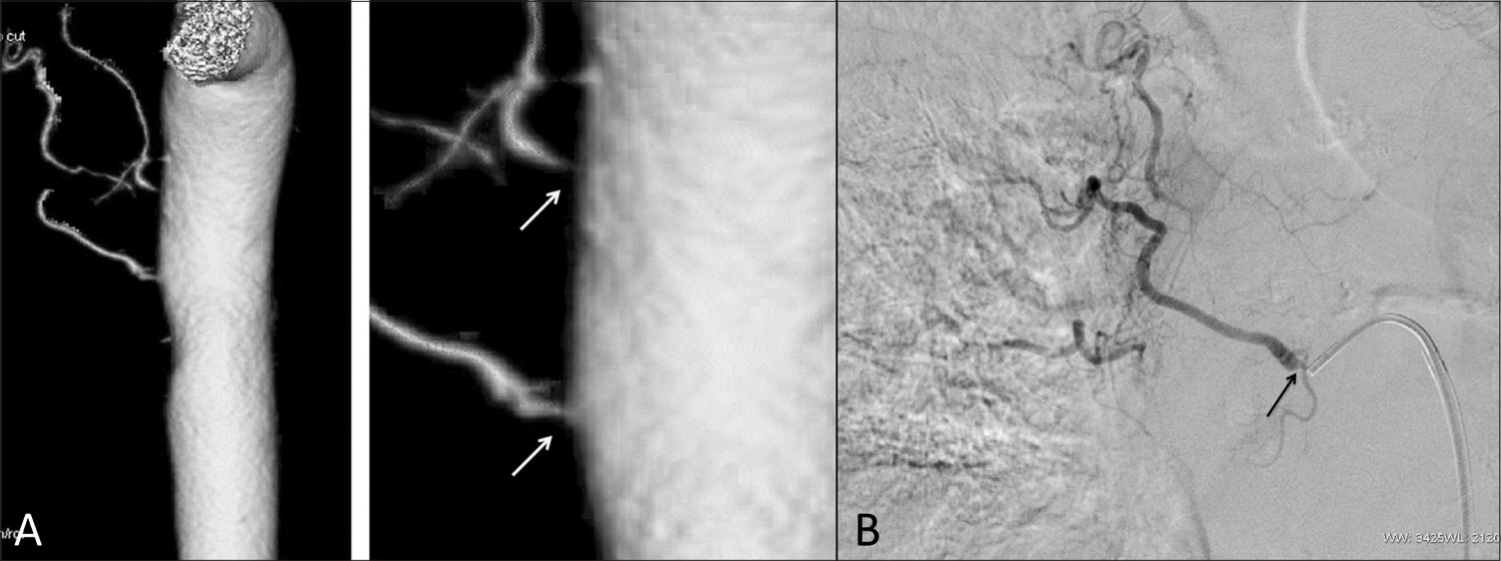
Bronchial artery angiograms. CT angiogram of the aorta (A) shows severe stenoses (arrows) at the ostia of the right bronchial arteries. DSA (B) shows severe ostial stenosis (arrow) in the culprit vessel that was not embolized at the first sitting
